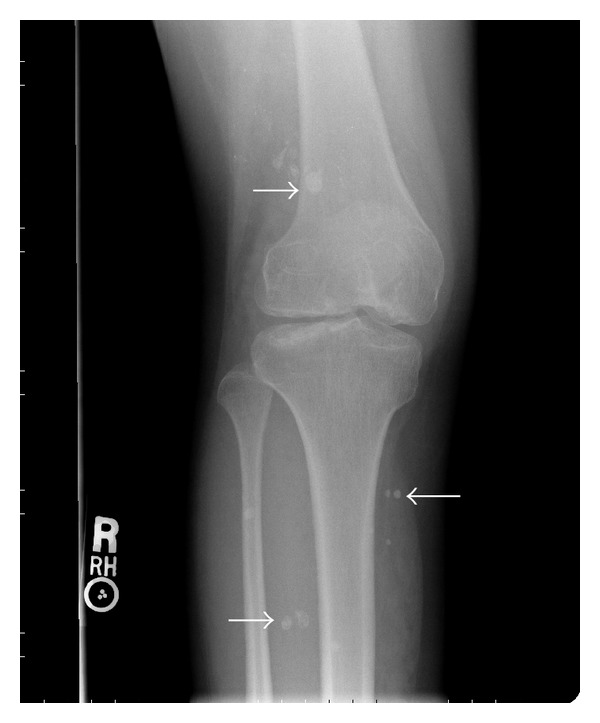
Anteroposterior radiograph of the knee demonstrating multiple venous calcifications (phleboliths) (arrows).
